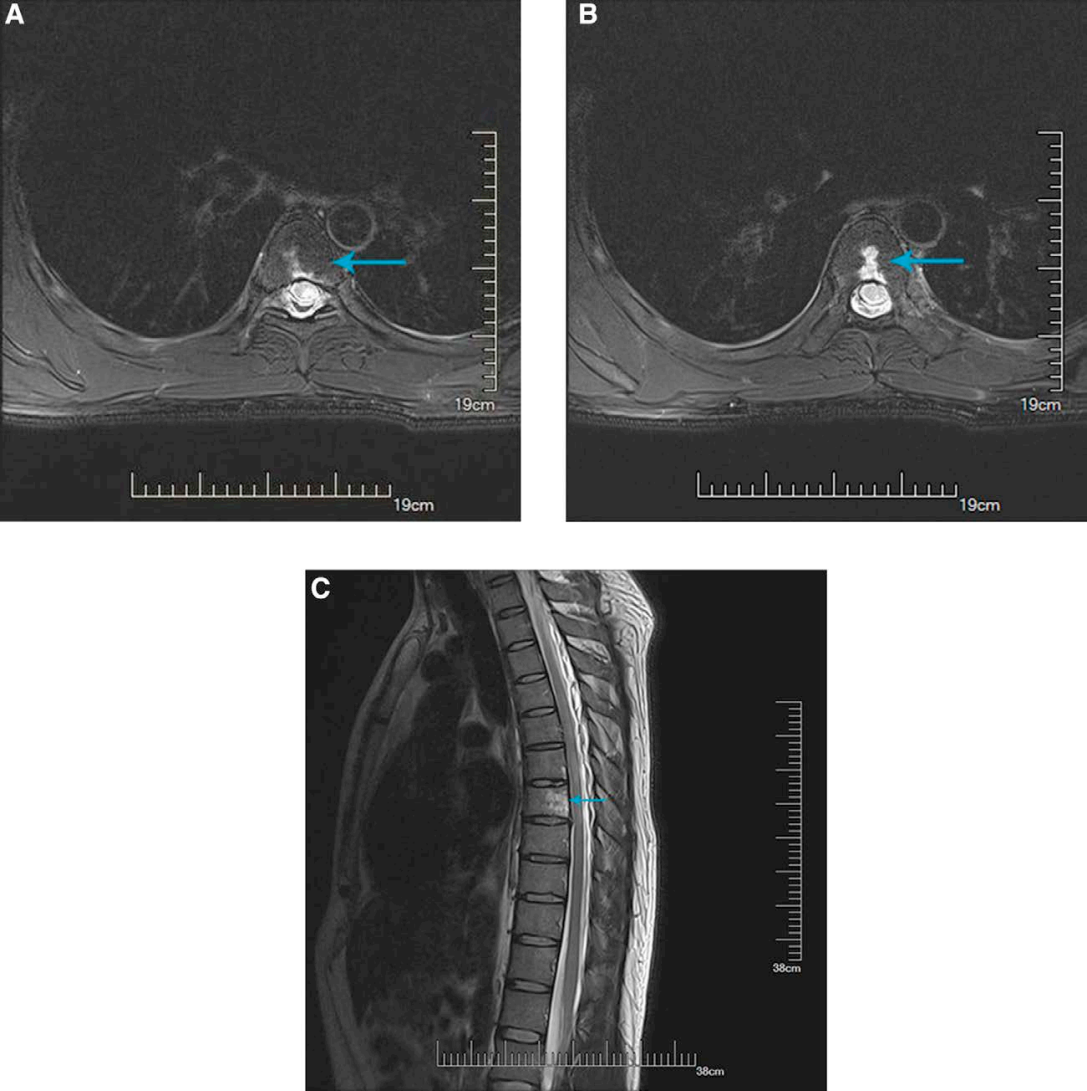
Axial MRI T2WI sequence showing high signal lesion in T7 spinal cord and vertebrates before treatment. MRI = magnetic resonance imaging, T2WI = T-2 weighted imaging.
